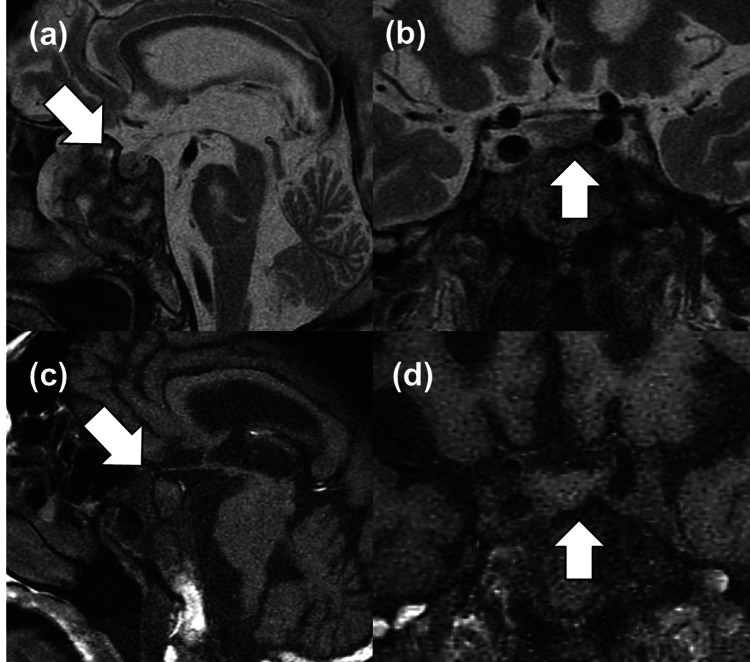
Images of the pituitary tumor Non-contrast coronal and sagittal T2- (a and b) and T1-weighted (c and d) pituitary images with MRI are shown. White arrows indicate a mass. MRI, magnetic resonance imaging
